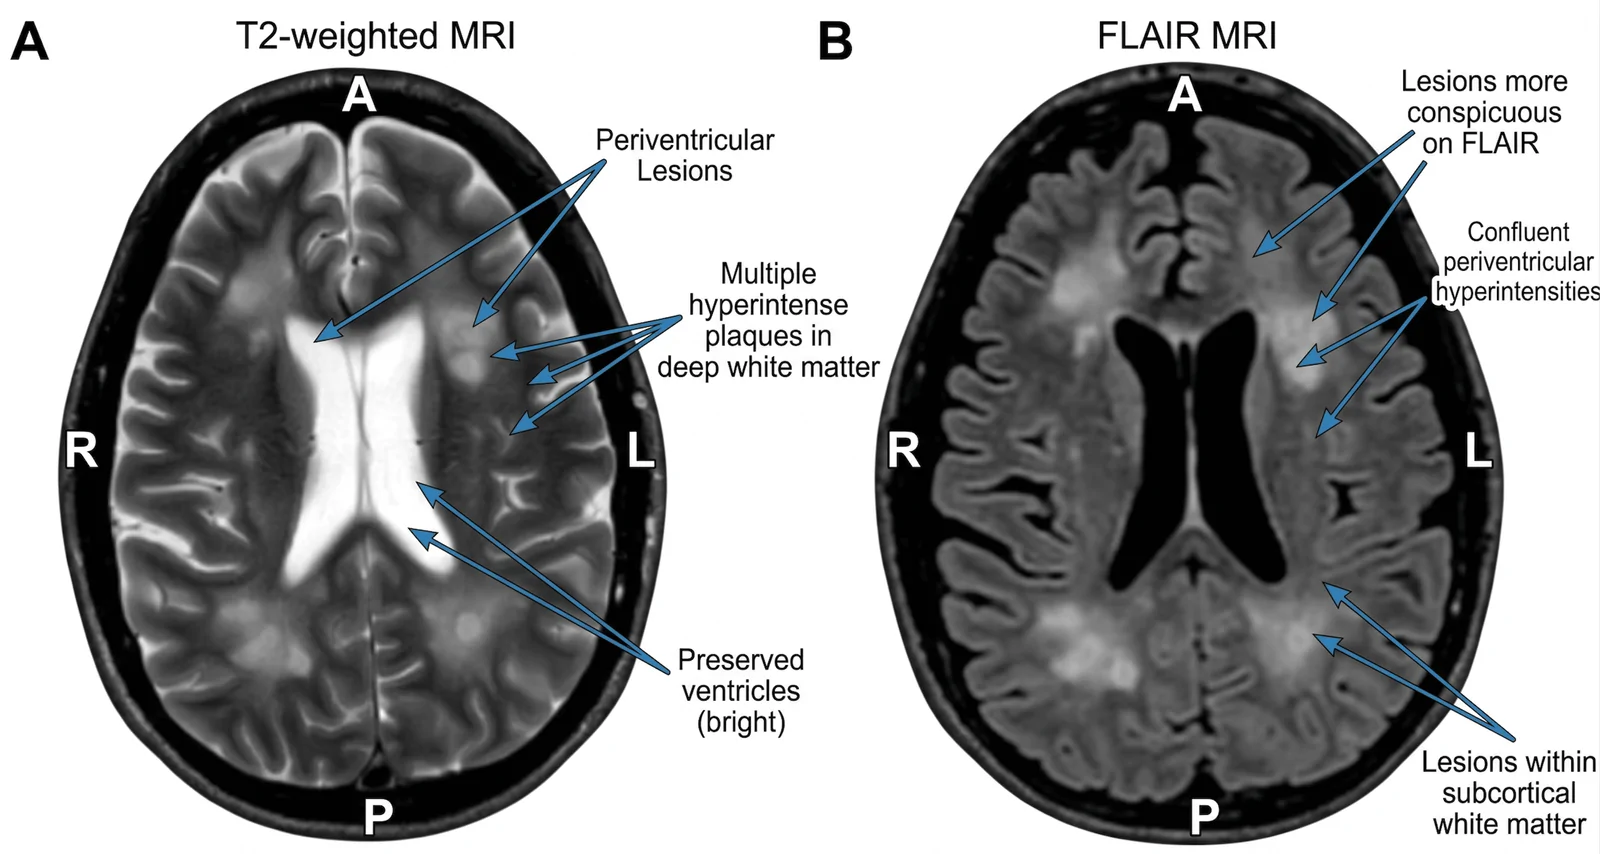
Axial T2-weighted (Panel A) and axial FLAIR (Panel B) MRI sequences demonstrate confluent, symmetrical periventricular, deep, and subcortical white matter hyperintensities consistent with diffuse toxic leukoencephalopathy. A: anterior; P: posterior; R: right; L: left; FLAIR: fluid-attenuated inversion recovery
